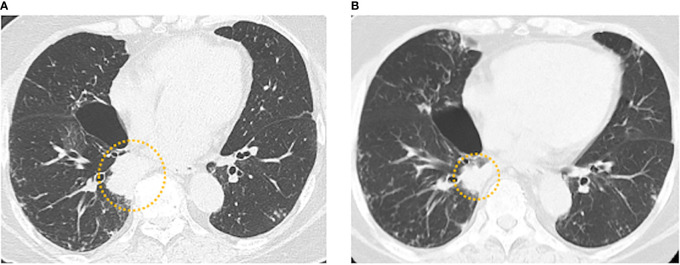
(A) Baseline chest computed tomography (CT) before tepotinib administration showing the tumor in the right lower lobe. (B) Chest CT 2.0 months after tepotinib administration showing a partial response (tumor shrinkage rate: 34.5% in diameter).
